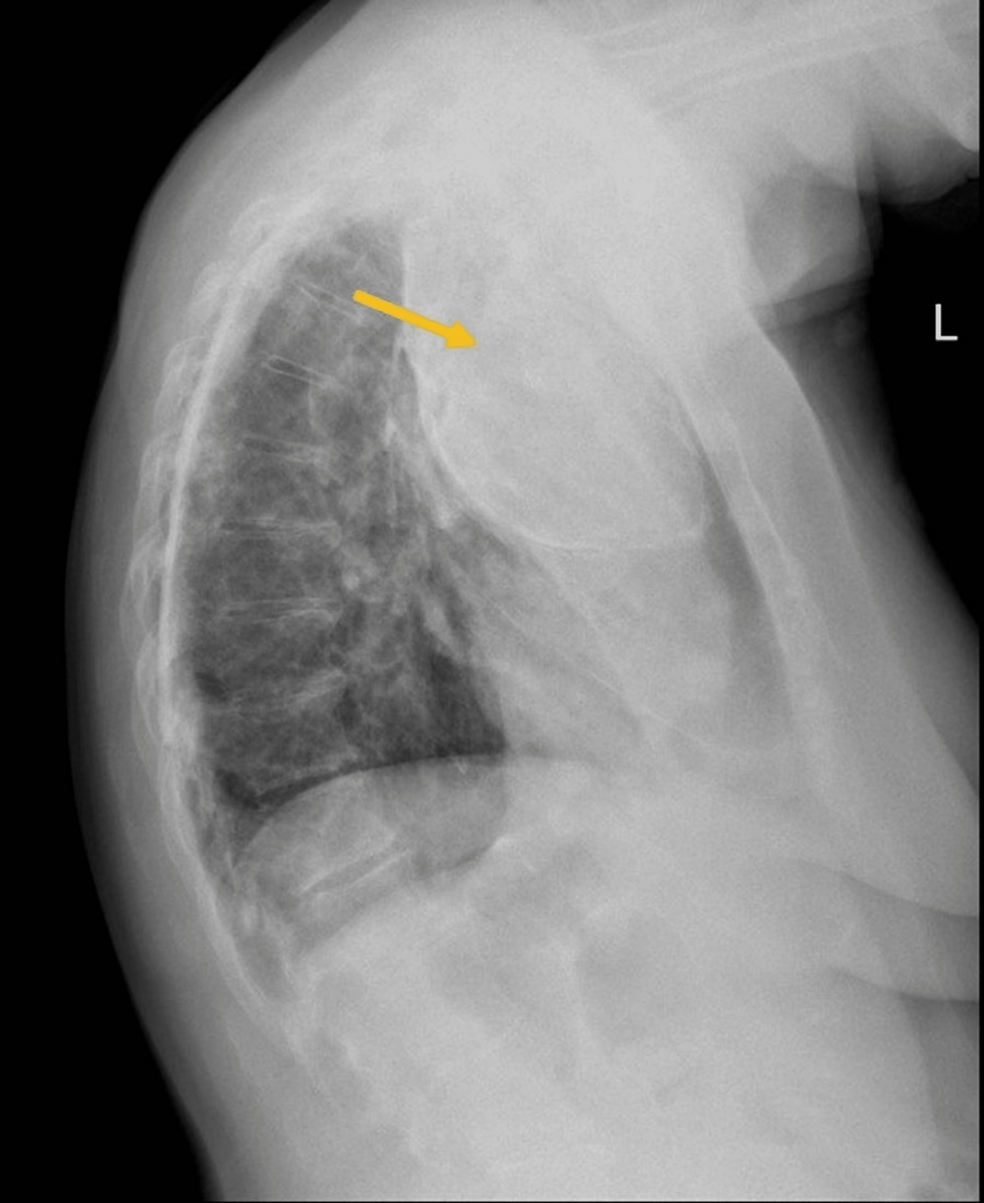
Lateral chest radiograph showing a calcified oleothorax (arrow)
